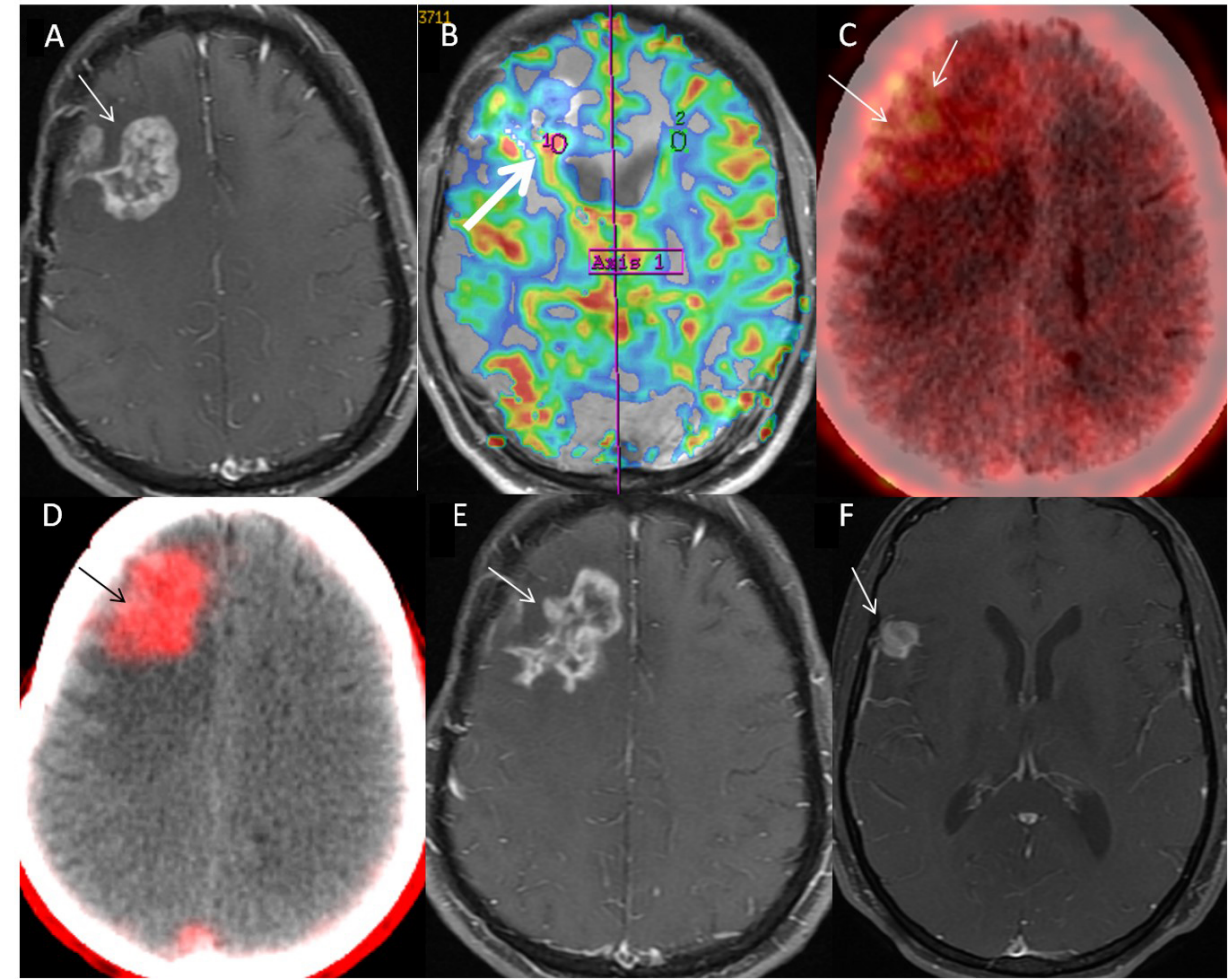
42-year-old male (patient 5) case of IDH-wild-type Grade IV glioma, of right fronto-parietal region, completed treatment in September 2021, presented with left-sided weakness in December 2021. Nodular enhancing lesion (Swiss cheese pattern) was seen on axial MR image (A, arrow), which showed a focal area of hyper perfusion with rCBV of 2.3 (B, arrow). This was attributed to predominantly post-treatment changes, BT-RADS 3B. FET PET shows patchy uptake in the lesion (C, arrow) with T-W of 4.0, despite the visual low-intensity of uptake. FAPI PET/CT image (D, arrow) showed well-defined area of intense uptake which corroborated with lesion on MR, with SUV_max_ of 3.6. Diagnosis of recurrence was established and patient was started on Bevacizumab. Follow-up MR at 3 months (E, arrow) showed mild reduction in extent of enhancement (pseudo-response to Bevacizumab) with a new enhancing lesion in right temporal region (F, arrow) suggestive of disease progression. rCBV: relative cerebral blood volume; FET: fluoro-ethyl tyrosine
